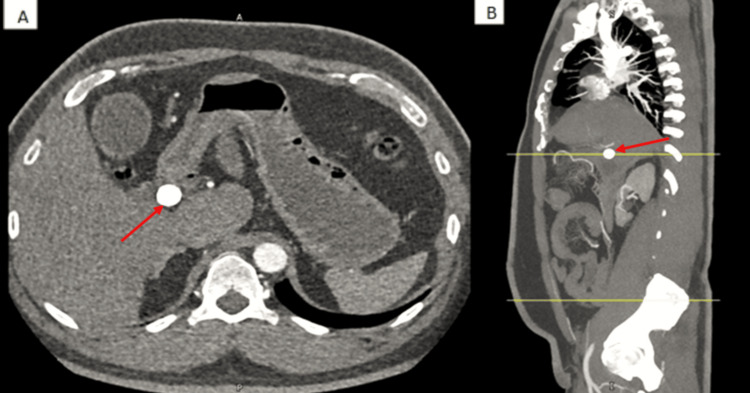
Non-contrast-enhanced CT scan showing a 1.7 cm gallstone impacted at the neck of the gallbladder (red arrows). (A) Axial view and (B) coronal view. There is gallbladder distension without pericholecystic fluid or biliary ductal dilation.
